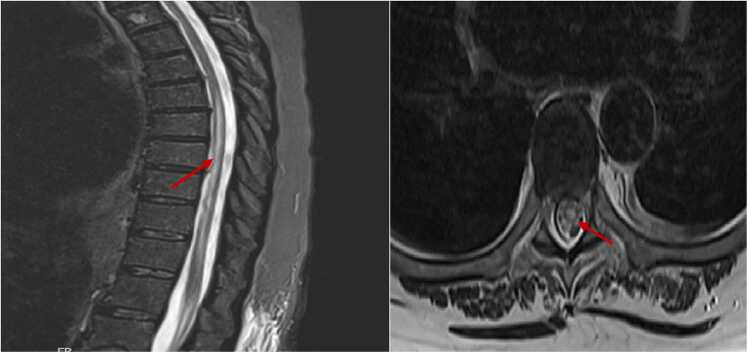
Sagittal (A) and axial (B) T2-weighted MRI of the thoracic spine demonstrate LETM. In panel A, the hyperintense signal (indicated by arrow) spans from T6 to T10 vertebral levels, consistent with a long-segment central cord lesion. Panel B shows a corresponding axial view with central cord hyperintensity, without evidence of significant cord swelling or mass effect.
